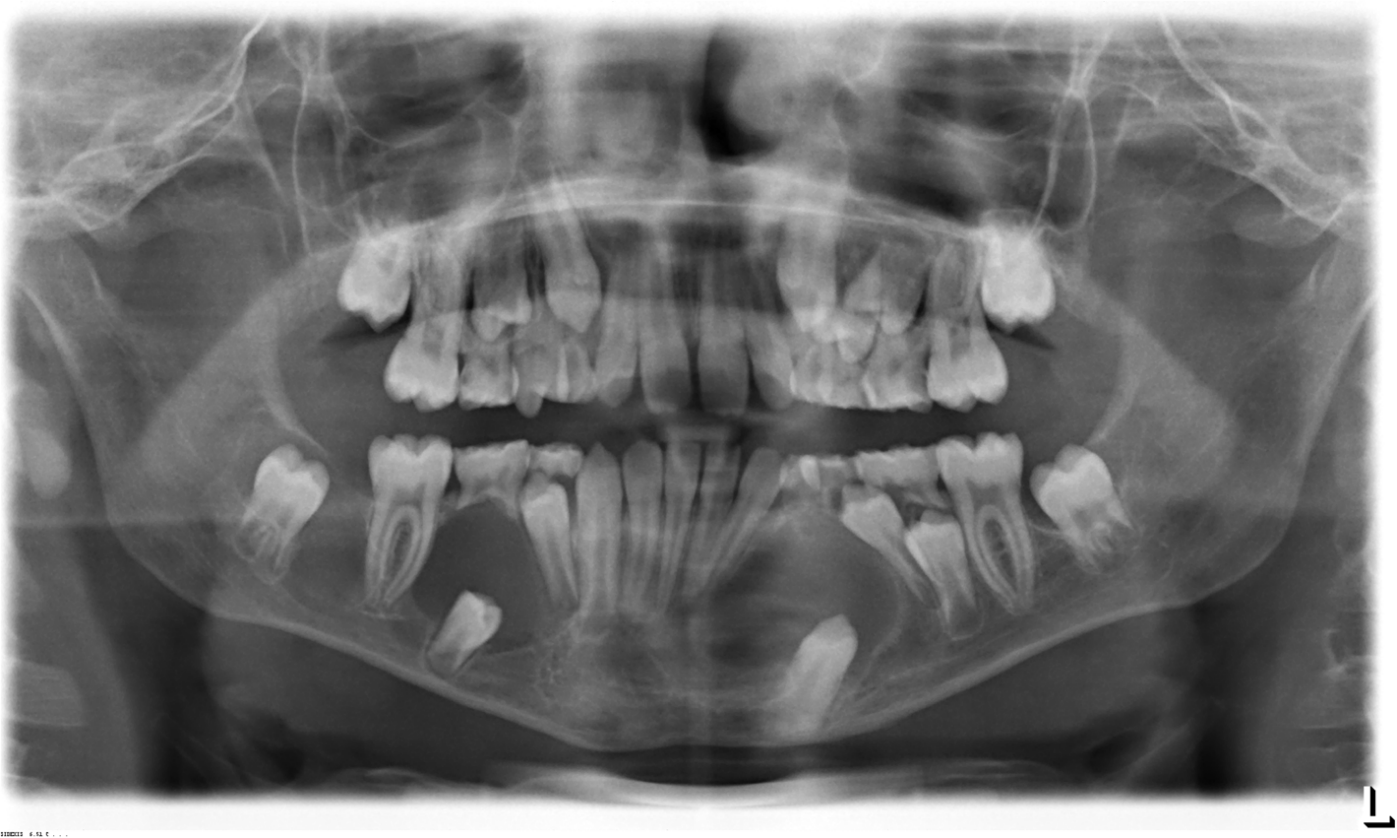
Initial orthopantomogram (OPT) at the age of 11 years showing the extended pericoronar osteolyses of the teeth 33 and 45 which were retained at the base of the mandible. The adjacent teeth (32, 34, 44) seem to be dislocated by the cystic lesions. In the area between teeth 46 and 47, a diffuse osteolysis confluent with a peri-coronar osteolysis around the retained 47 is obvious. Furthermore, root resorptions at the teeth 73 and 85 are visible
